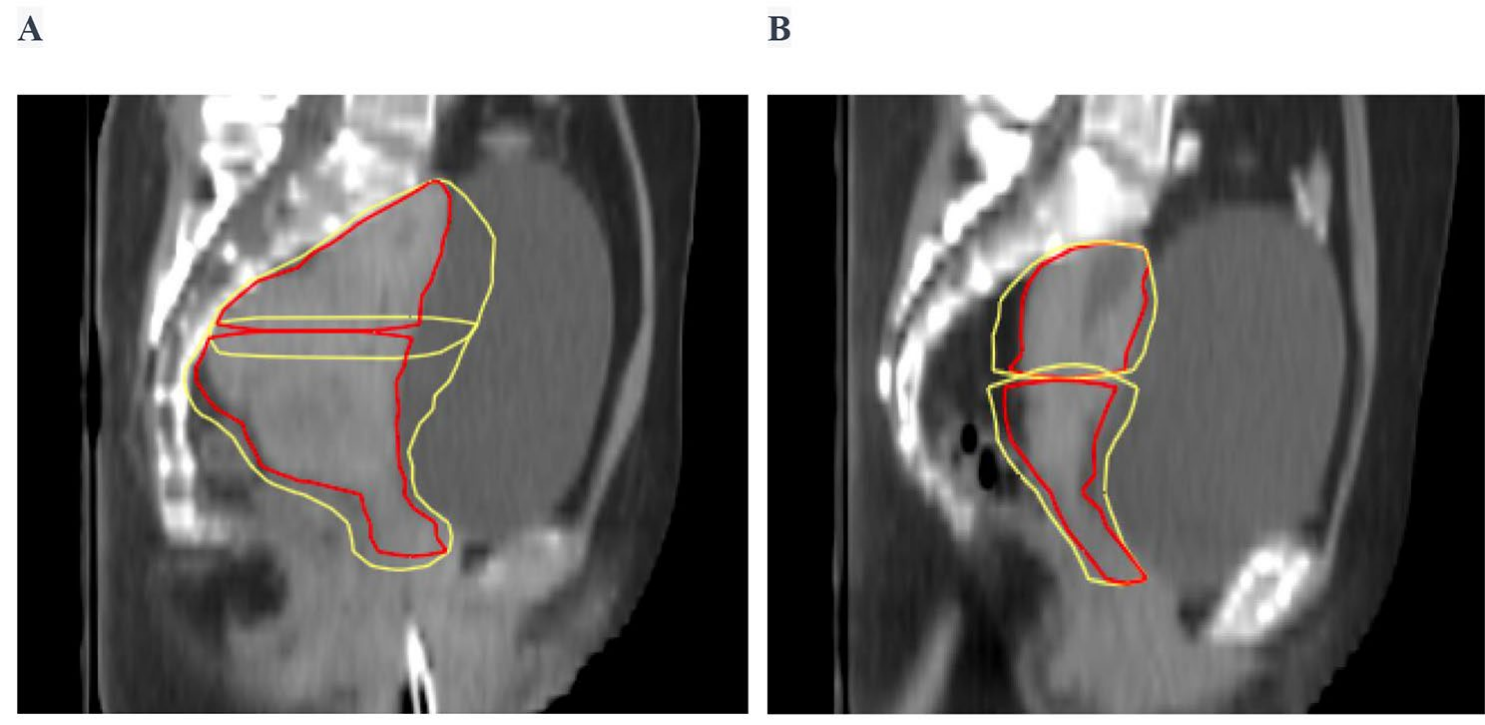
Sagittal slices of a planning CT scan from different patients. Daily clinical target volume contours from the iCBCT were mapped to the planning CT after registration with respect to bony anatomy. Initial CTV-U and CTV-C are shown in red, ultimate CTV-U and CTV-C are shown in yellow
